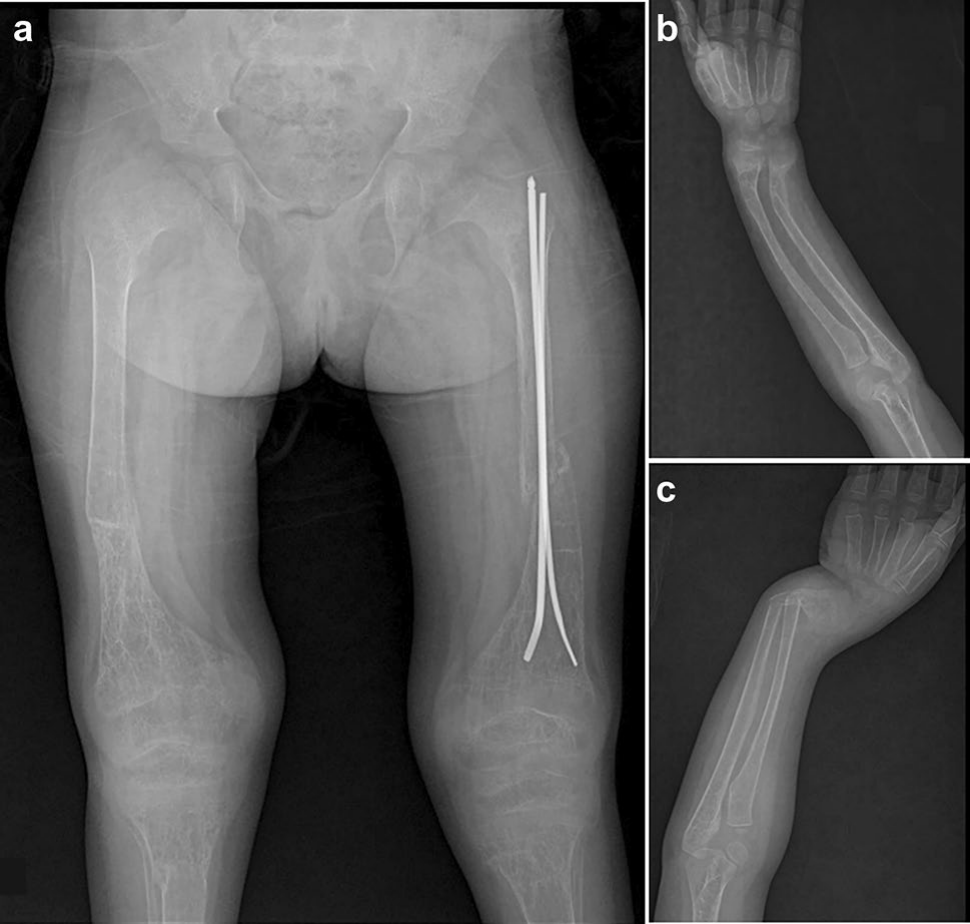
Radiographic profile of P10 (age 8 y) with Fanconi–Bickel syndrome. Multiple sites of fracture and osteopenia are seen in her upper and lower limbs (a–c)
